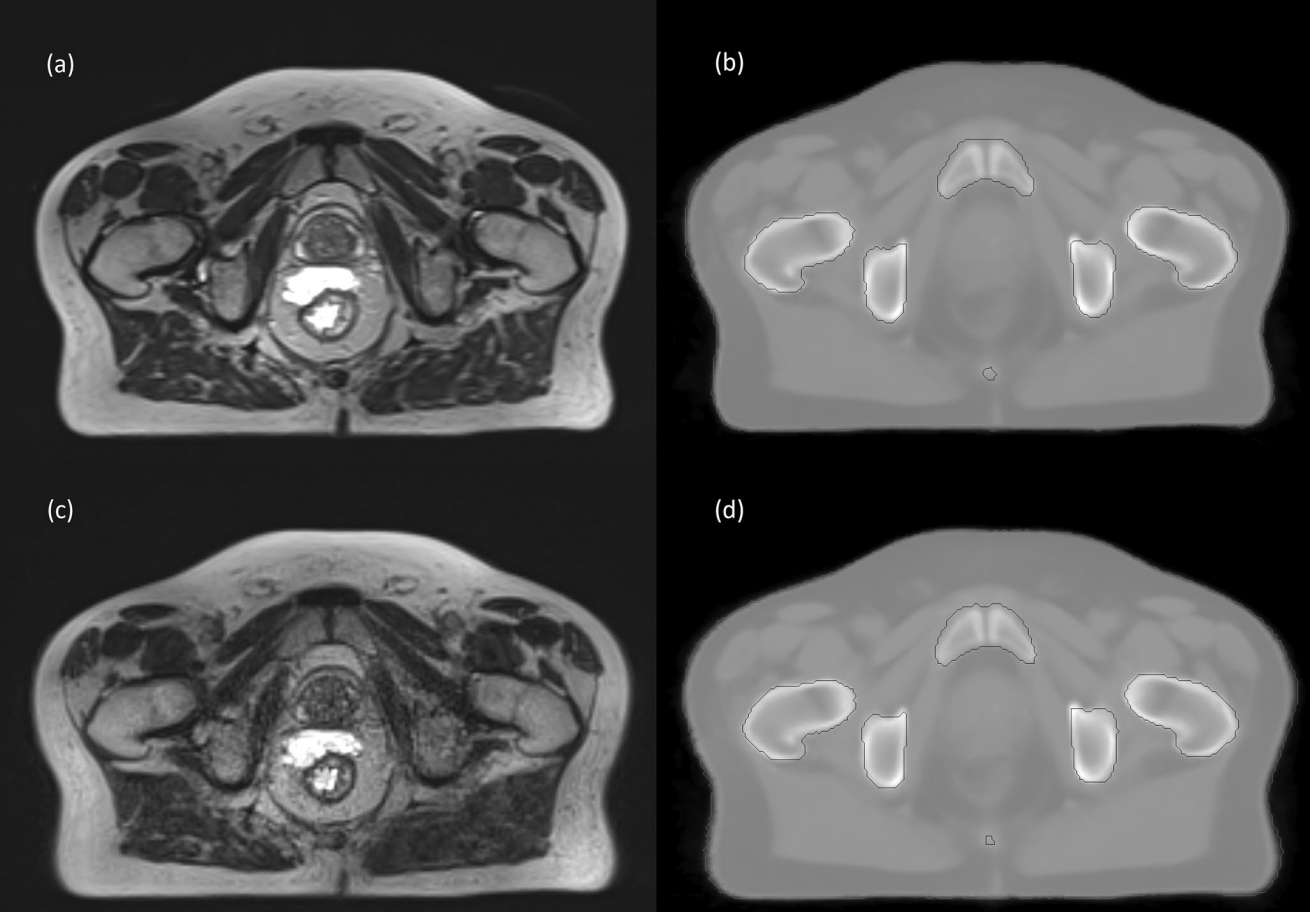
An example of the (a) standard MRI and (b) S-sCT and the (c) fast MRI and (d) F-sCT for the same corresponding slice for one patient. The body and bone masks are also displayed on the sCT slices in (b) and (d)
